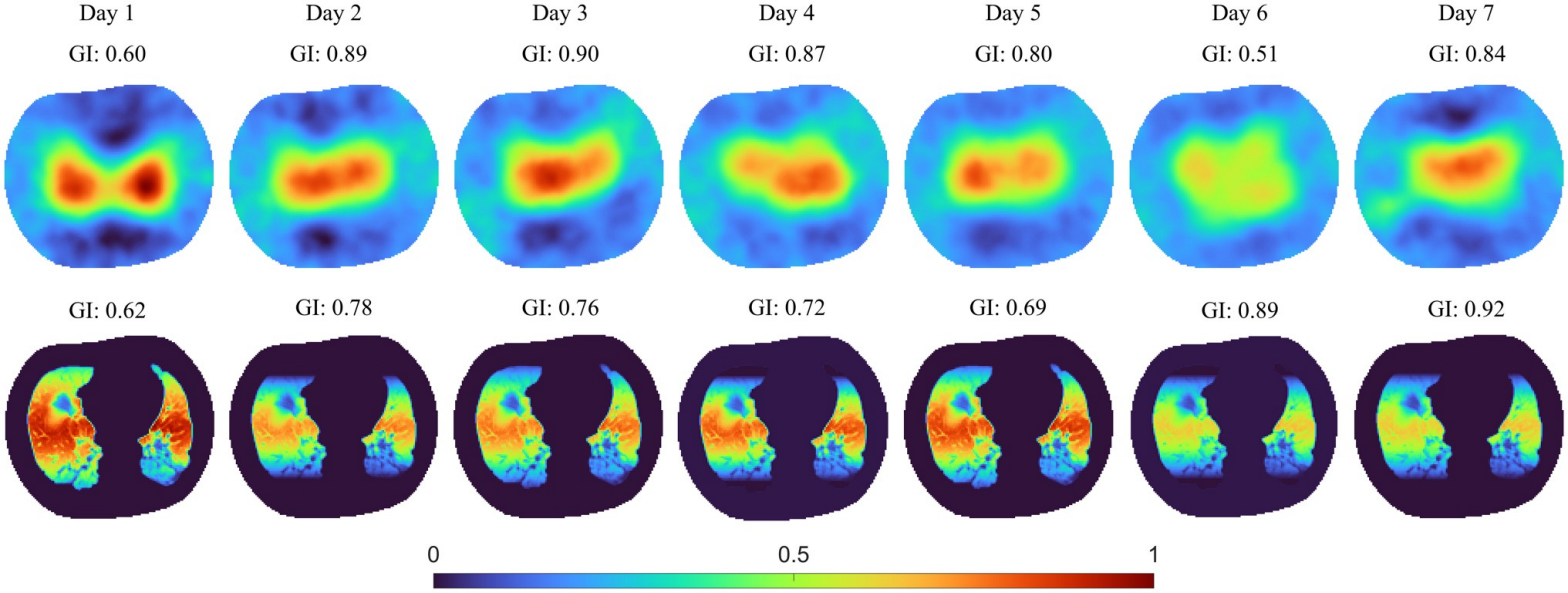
A seven-day monitor procedure of the patient A at the PEEP stage of 25 cm H_2_0. GI indices were calculated and provided above each image. First row: GREIT reconstructions of conductivity change; second row: Reconstructions of conductivity change using the DCT-based EIT approach with detail information prior derived from the related CT image.
